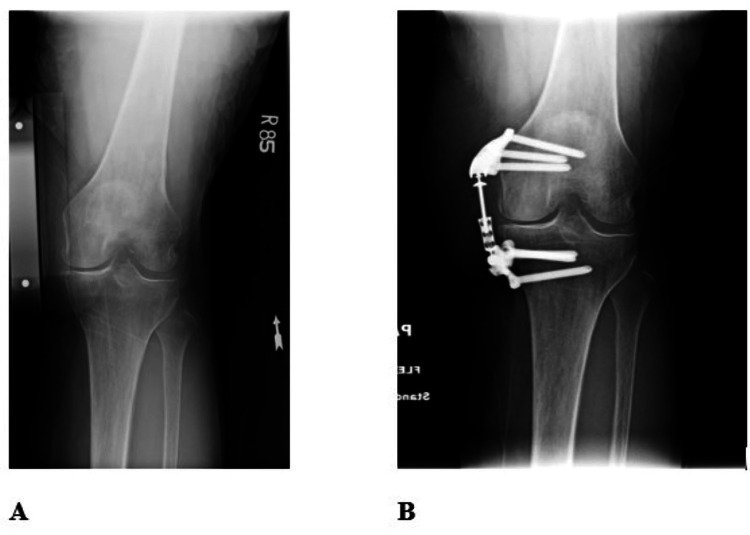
Preoperative posteroanterior (PA) radiograph (A) compared with the five-year postoperative radiograph (B) Imaging indicates preservation of joint integrity, implant stability, and no signs of loosening or hardware failure at five years post procedure.
